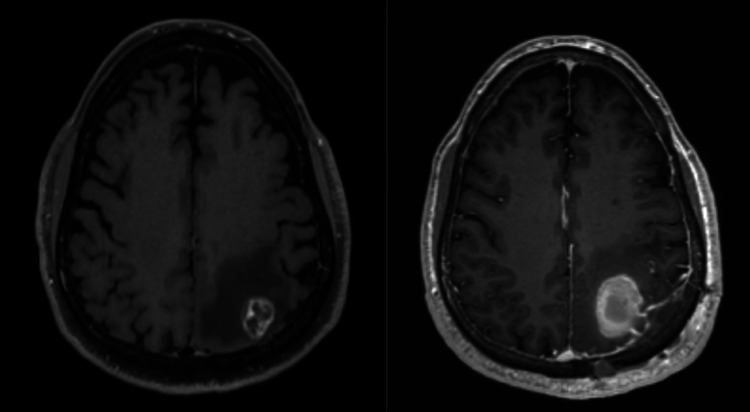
T1 MRI of the patient Preoperative T1 + gad revealing a left parietal 2-cm peripherally enhancing mass (left), and postoperative T1 + gad revealing hemorrhage into the tumor bed with no residual tumor (right) MRI: magnetic resonance imaging; gad: gadolinium
